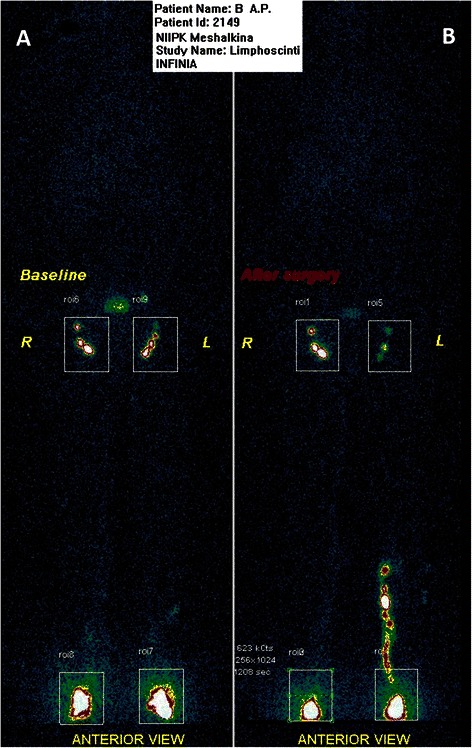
Lymphoscintigrams: before the surgery (а) and on Day 7 after the open vein harvesting on the left lower limb (b)
